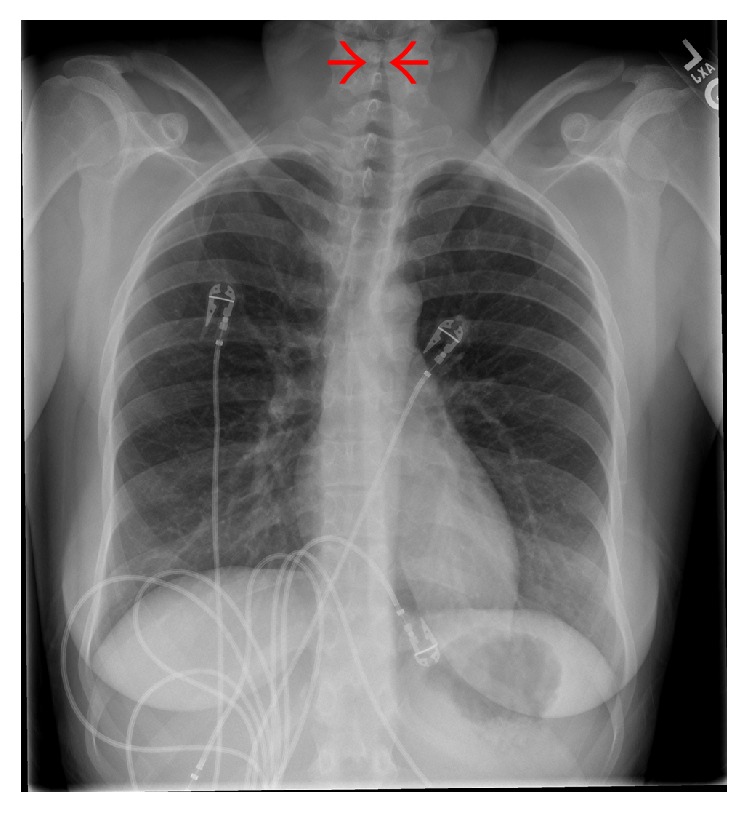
Hospital day 0 posterior-anterior chest radiograph demonstrating subglottic stenosis, known as Steeple sign (between red arrows).
